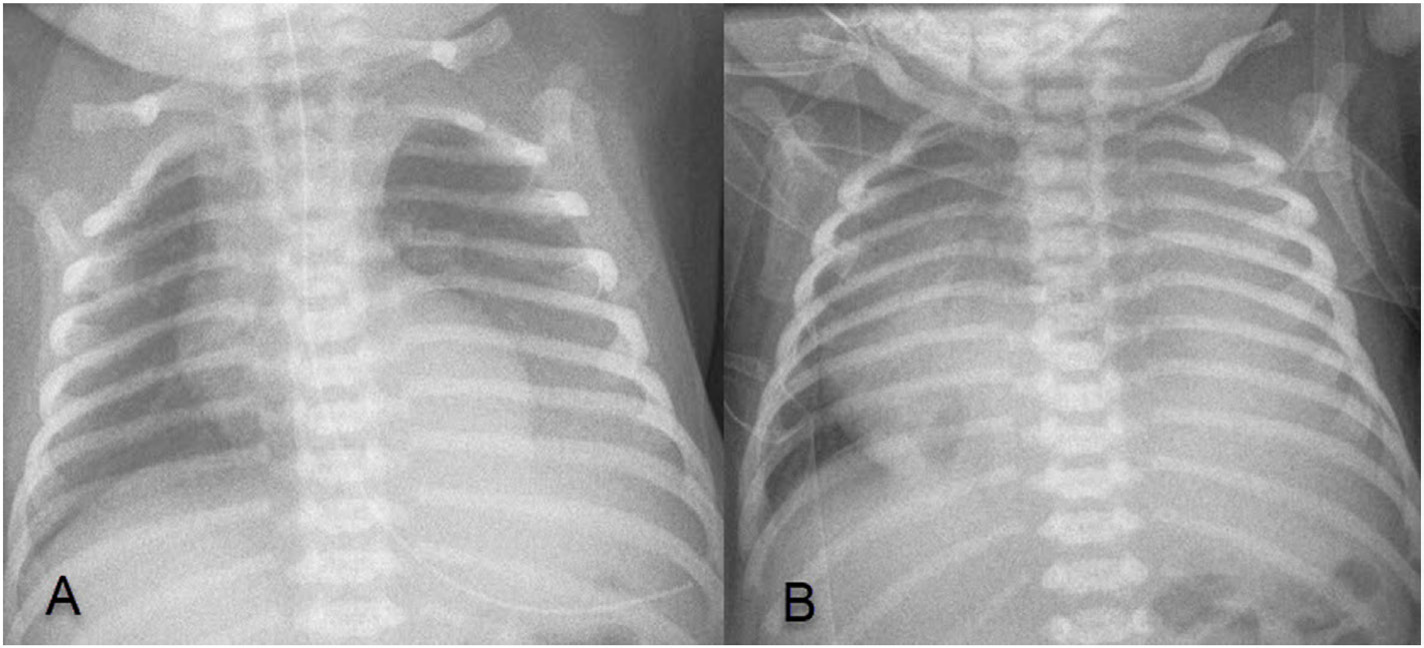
(A) X-ray 4 h after birth showing left basal thoracic mass, without significant pleural effusion (PE); (B) X-ray at 18 h of age showing a massive left sided PE causing mediastinal shift.
